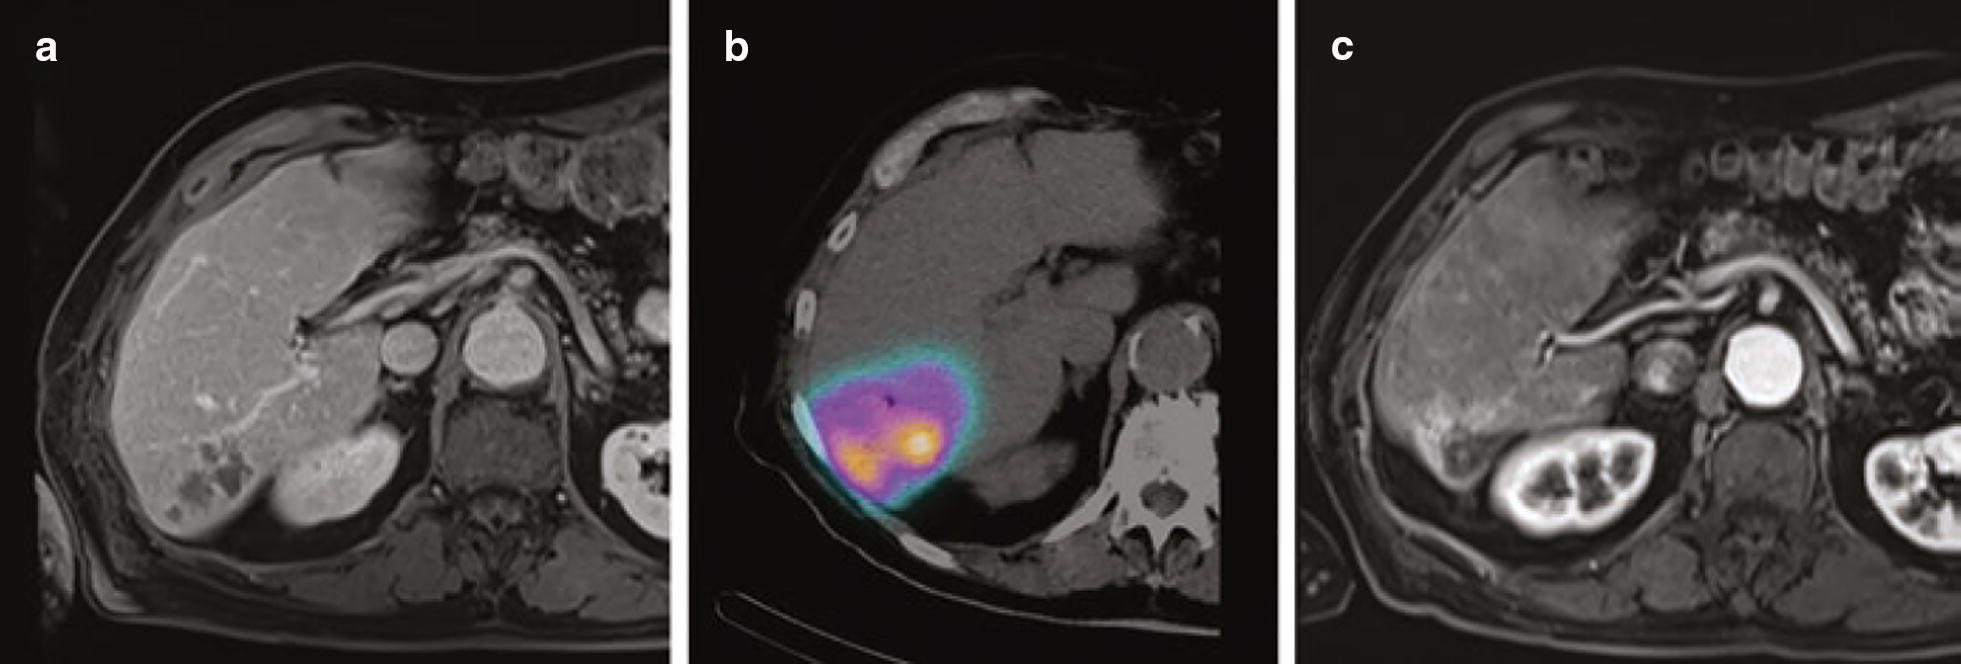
Superselective segmental radioembolization. a CT scan before treatment showing multinodular tumor. b PET scan showing intense radiation after administration of ^90^Y resin microspheres. c Significant atrophy of liver segment and lack of tumor activity 1 year after treatment (this figure is reproduced from Sangro et al. [253] with required copyright permission)
